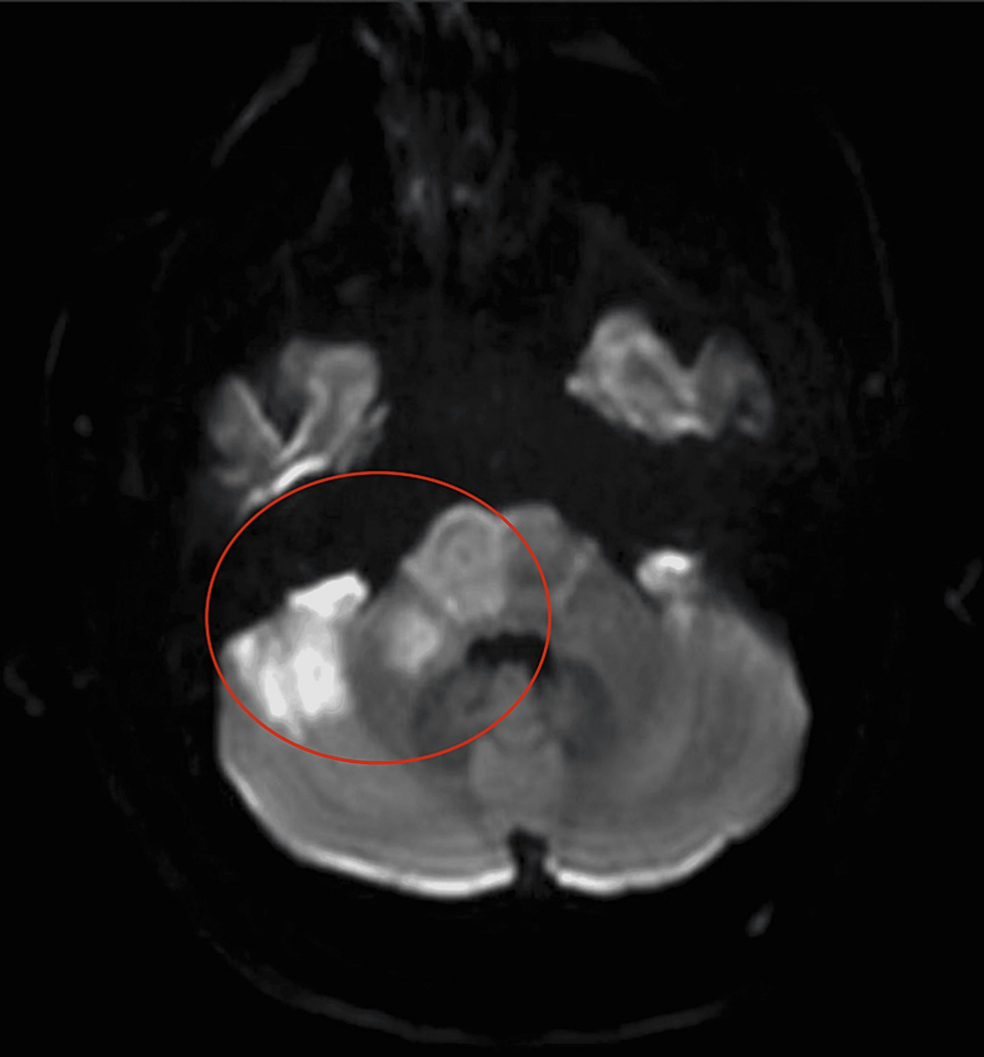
MRI brain without contrast showing ischemia/infarction within the right midbrain, right pons, right brachium pontis, and right cerebellar hemisphere (circle). MRI, Magnetic Resonance Imaging
